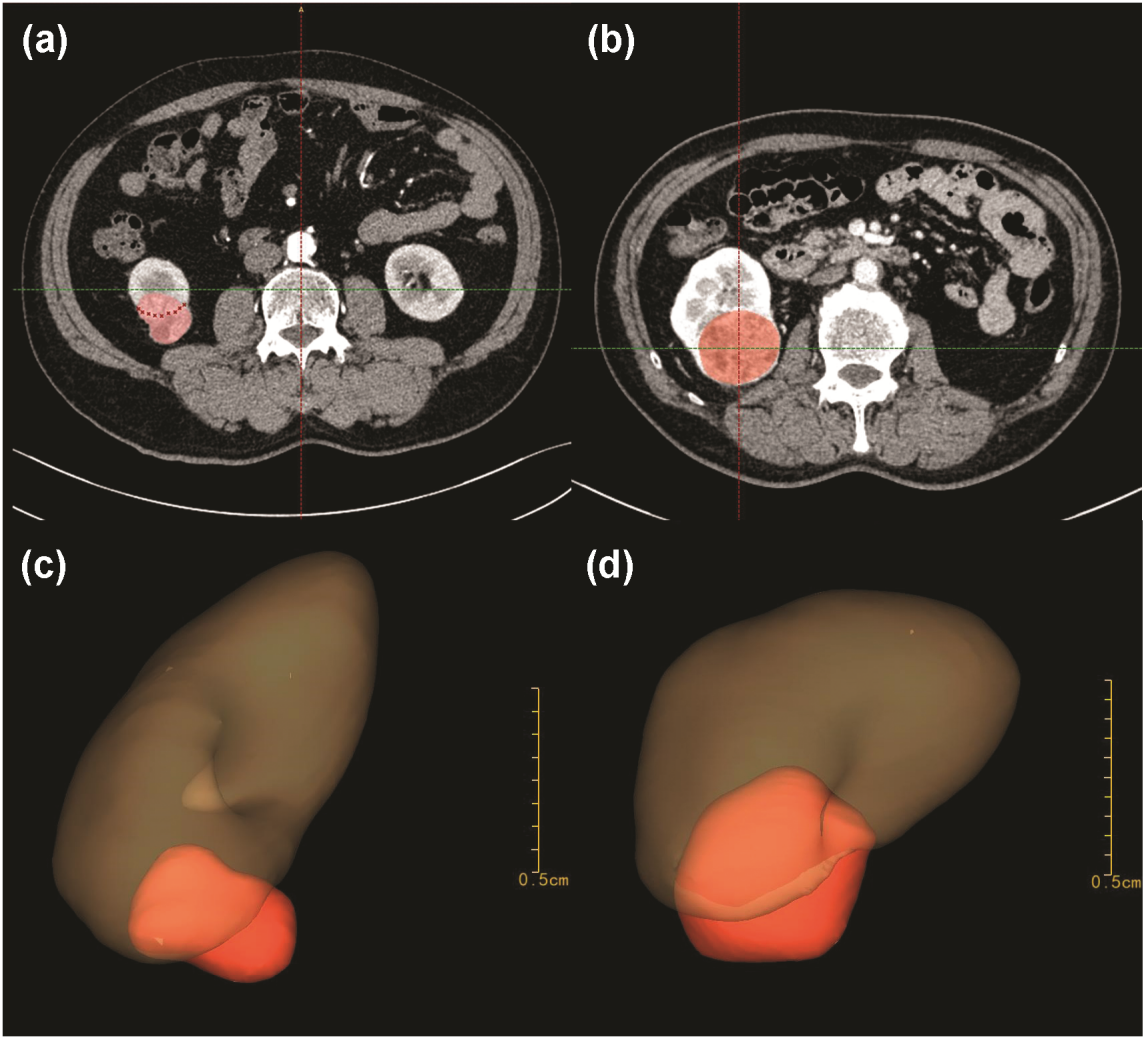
Inconsistency of endophytic-percentage between 2D and 3D dimensions. Patient C in Fig. 4 measured a <50% endophytic characteristic in traditional 2D CT images (A). But the patient measured a >50% endophytic characteristic in 3D models (C). Patient D in Fig. 4 presented an entirely-endophytic characteristic in 2D cross-section, which section showed the tumor’s longest diameter (B). After 3D reconstruction, the same patient presented a >50% endophytic characteristic in 3D models (Fig. 4D).
